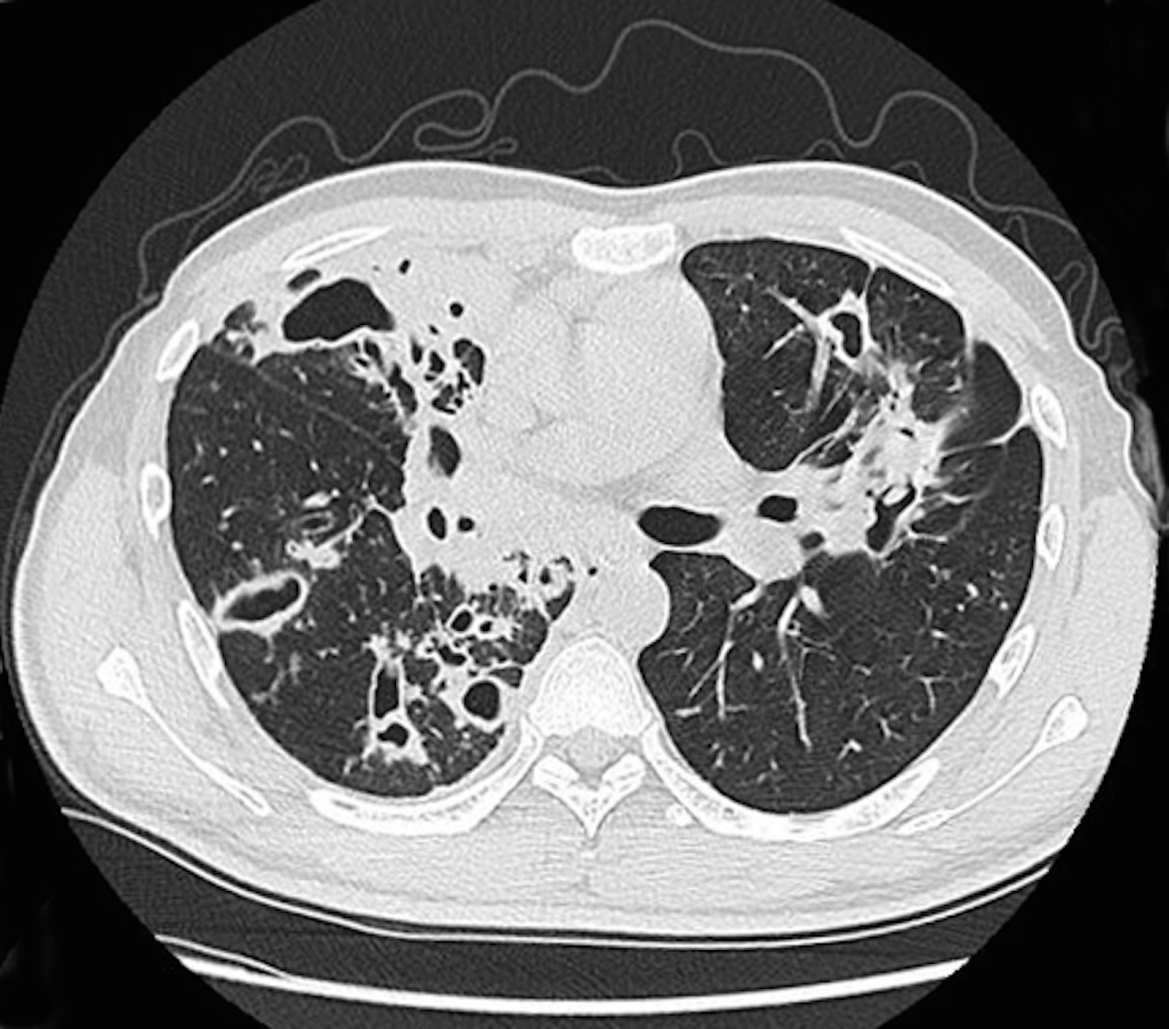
Primary multidrug-resistant TB in a 29-year-old man. A lung window of a transverse thin-section CT scan (1.25-mm-section thickness) showing multiple cavities in both lungs.
